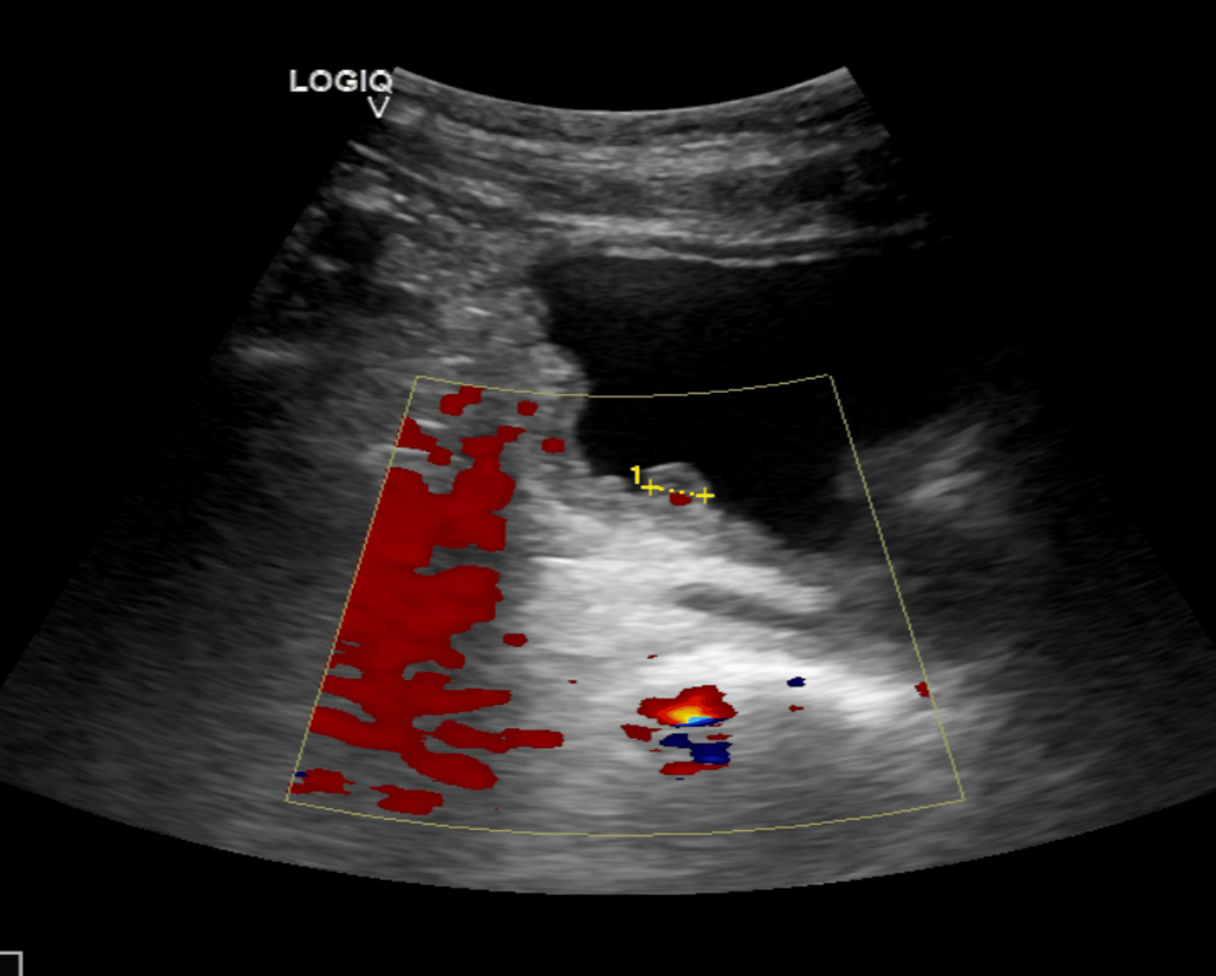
Ultrasound of the bladder. Doppler imaging showing internal vascularity of the 2.5 cm³ lesion.
